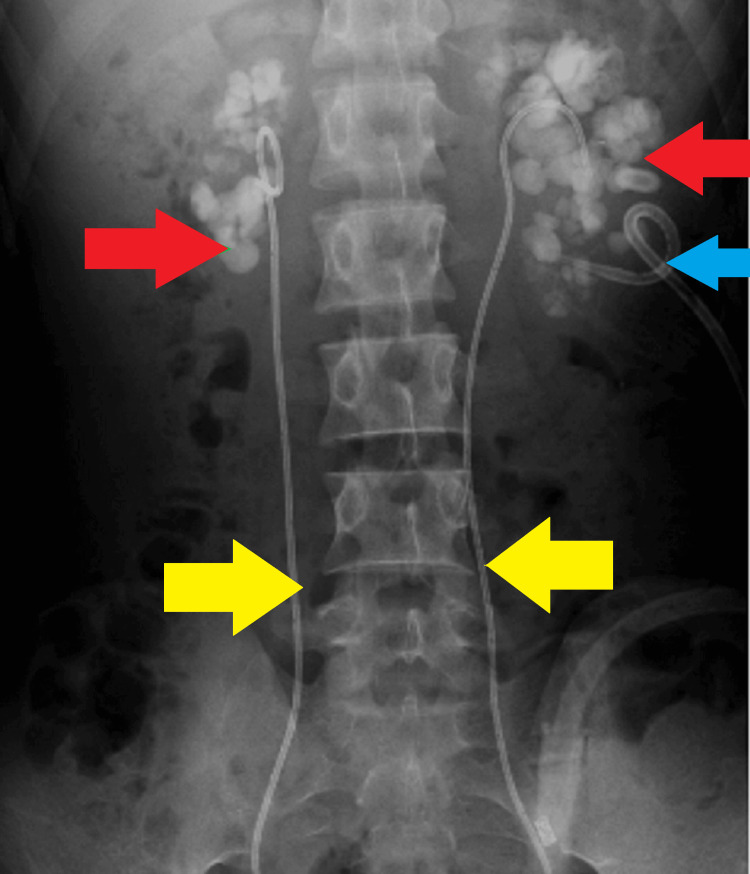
X-ray KUB X-ray KUB showing bilateral staghorn calculi (red arrow) with bilateral DJ stenting (yellow arrow) and left PCN (blue arrow). KUB: Kidneys, ureter, and bladder; PCN: Percutaneous nephrostomy
